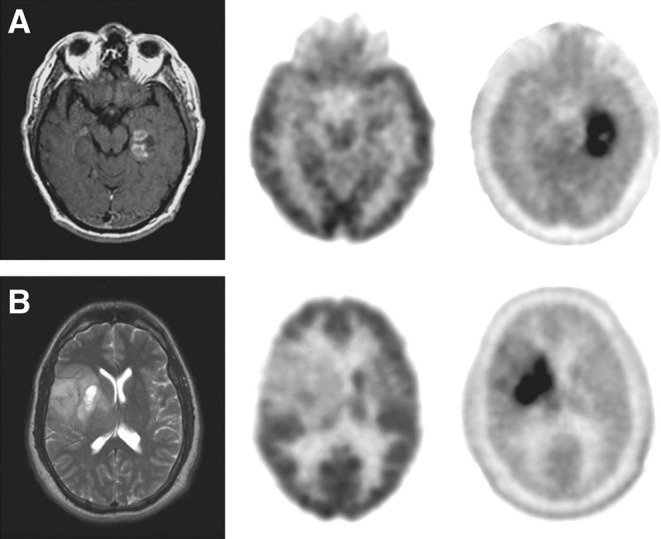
MRI (left), ^18^F-FDG PET (middle), and ^18^F-FDOPA PET (right) of newly diagnosed tumors. (A) Glioblastoma. (B) Grade II oligodendroglioma. This research was originally published in Chen et al. (95).
